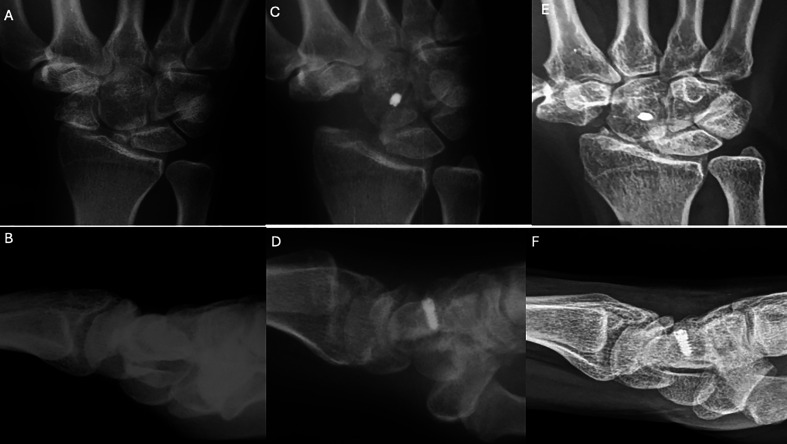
Clinical case of FCR tenodesis over capitate (Ligamentous grip) and Scaphoid excision. A and B. Preoperative wrist X-rays of SNAC stage II (AP and Lateral views). C and D. Postoperative wrist X-rays (AP and Lateral views) after the removal of K-wire (1month). E and F. Postoperative wrist X-rays (AP and Lateral views) after 12.25 years. Source: Dr. Emmanouil Apergis.
